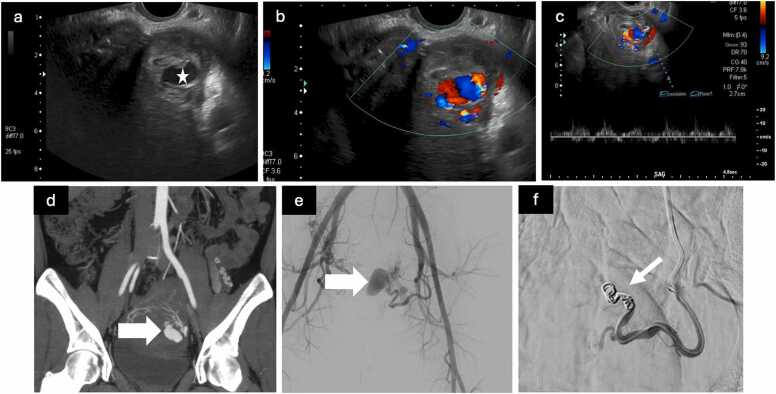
30 year– old female post C-section day 10 with postpartum heavy hemorrhage, tachycardia, and hypotension requiring blood transfusion. Transvaginal ultrasound (a) gray scale displays heterogenous myometrium with a central anechoic lesion (star) (b, c) Doppler interrogation shows localized myometrial vascularity with bidirectional flow pattern; “yin-yang” or “Pepsi” sign (d) Coronal CT pelvis maximum intensity projection (MIP) image and (e) Digital subtraction angiography image demonstrate a left uterine artery branch pseudoaneurysm (bold arrows) with (e) coil embolization (thin arrow). DIAGNOSIS: Uterine artery pseudoaneurysm.
